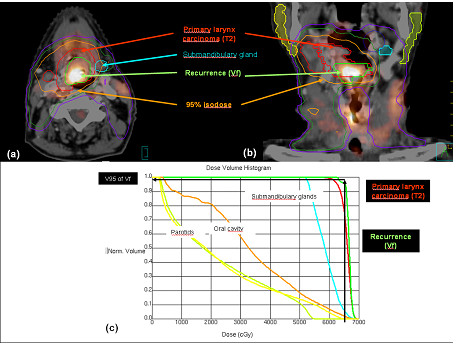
Example of in-field recurrence. The planning CT has been registered with the FDG-PET-CT imaging of the recurrence. The failure volume (*Vf) *appears fully within the 95% PTV isodose (a and b). The *Vf*-V95 is equal to 99.9% (c).
